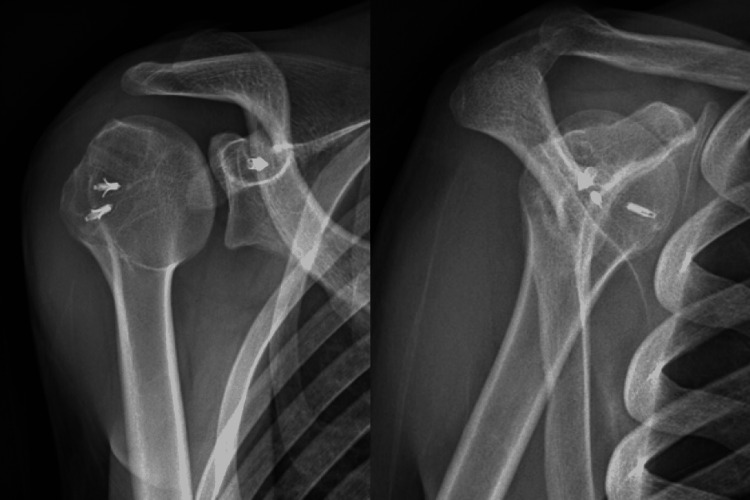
X-ray showing a congruent shoulder joint
